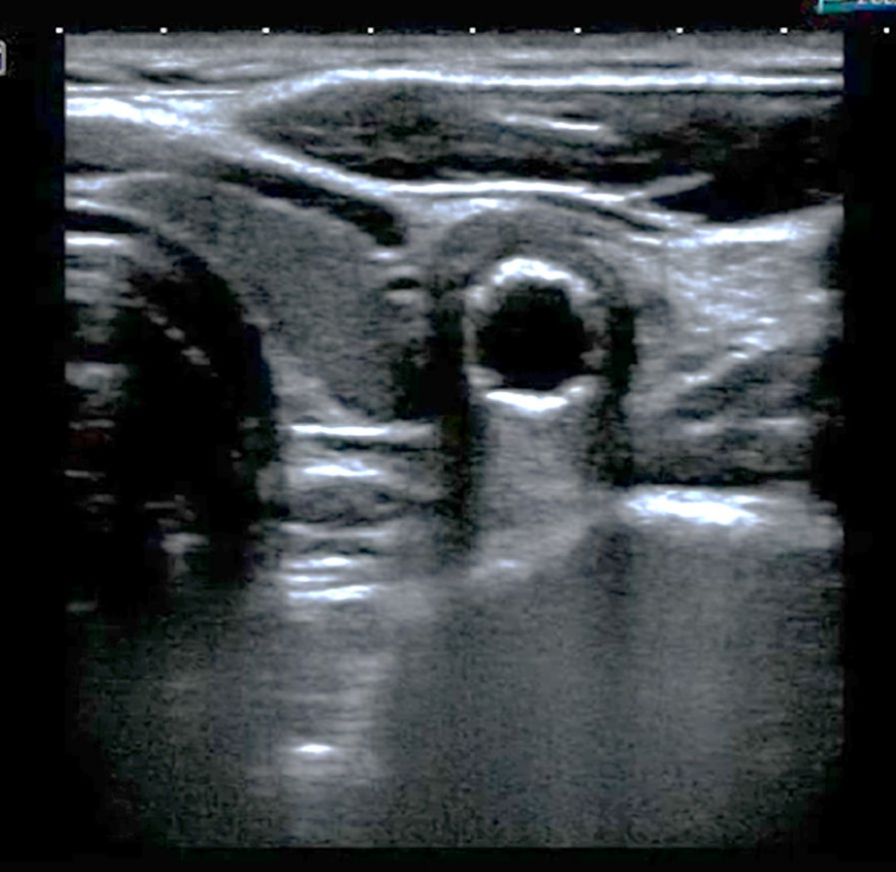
Ultrasound of carotid after stenting: Transverse view
